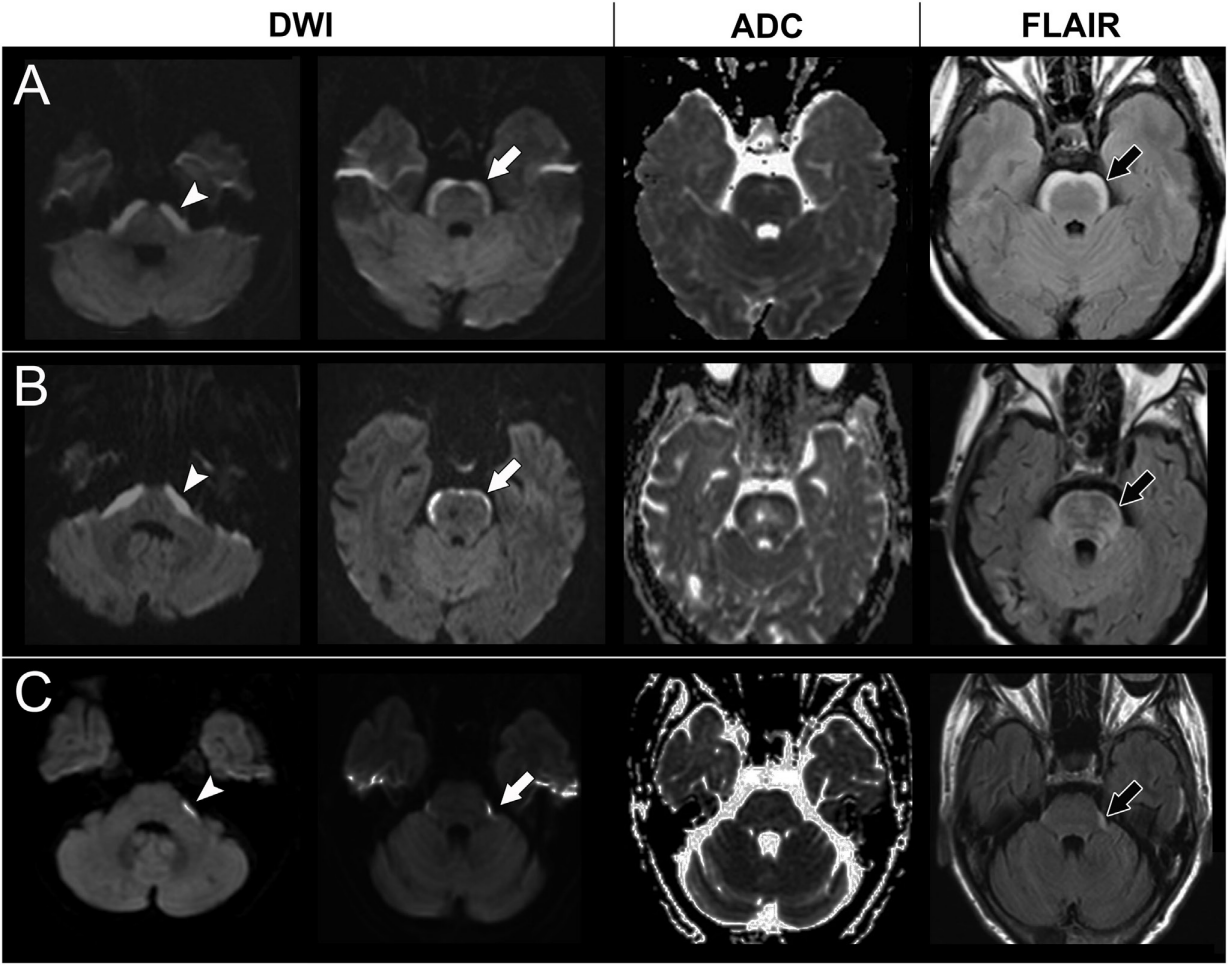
Brain magnetic resonance imaging of case 2, 3, and 4. A high signal in diffusion-weighted imaging (DWI) at the lower pons level (arrow head) and upper pons level (white arrow) with a corresponding low signal on apparent diffusion coefficient (ADC) mapping and hyperintensities on fluid attenuation inversion recovery (FLAIR) imaging (black arrow) in case 2 (A), case 3 (B), and case 4 (C).
